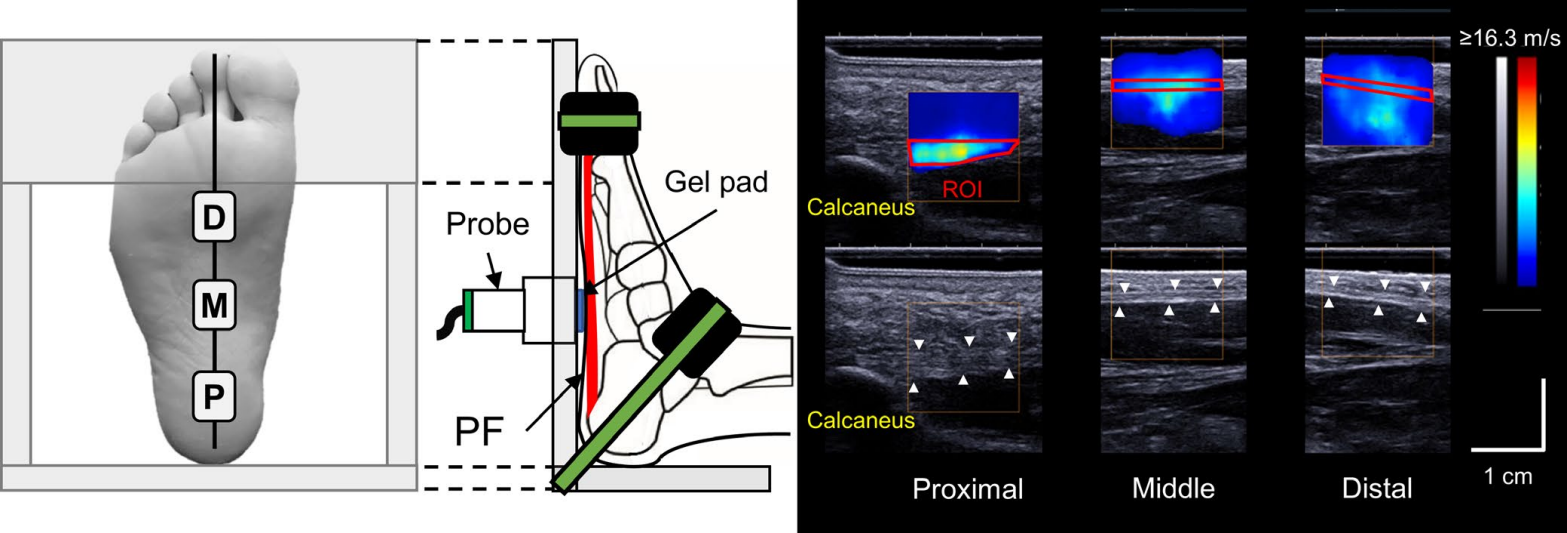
Experimental setup and representative ultrasound B‐mode and shear wave images of the plantar fascia (PF) at the proximal (P), middle (M), and distal (D) sites. ROI, region of interest
